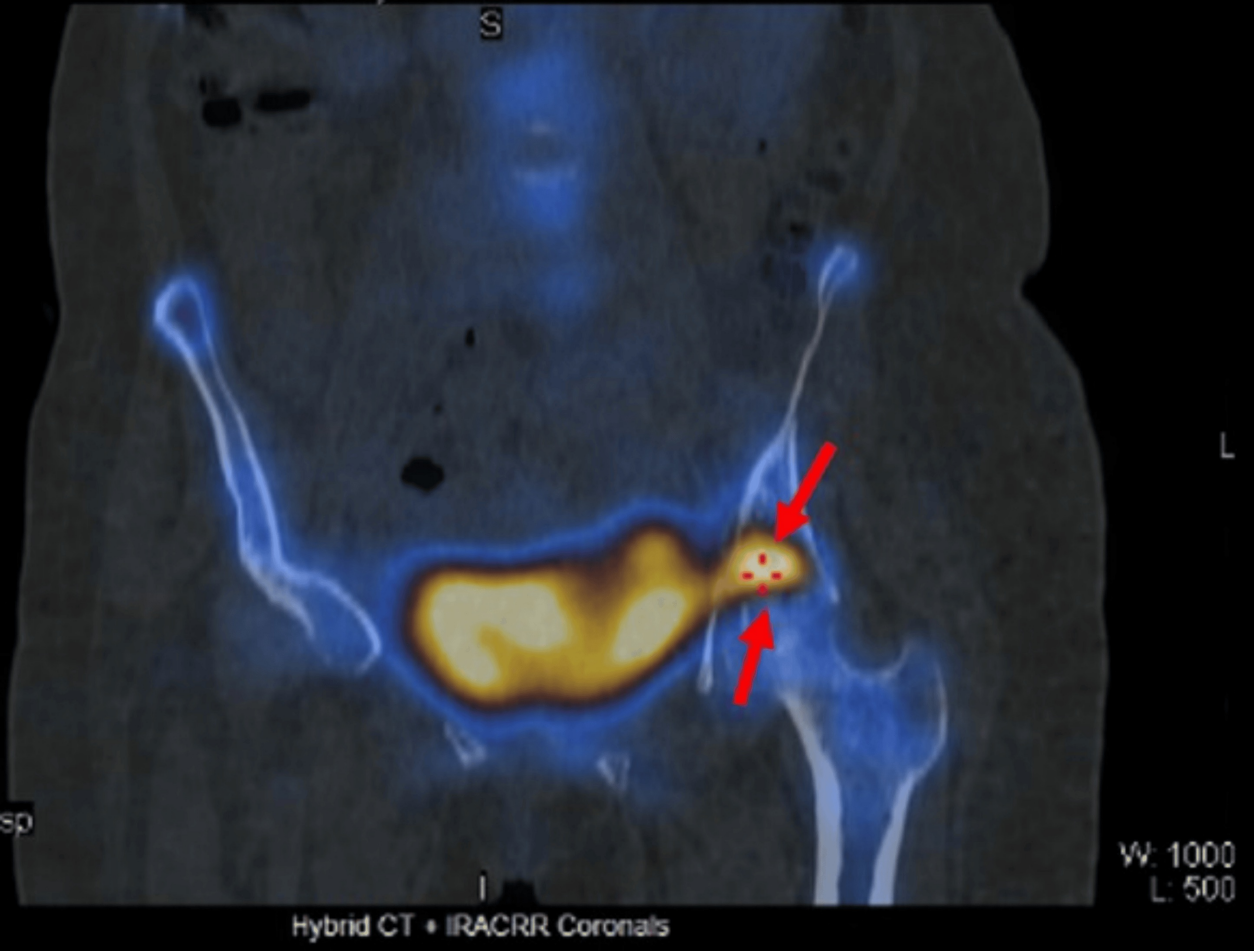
NM bone scan (coronal view) demonstrating a large osteoblastic metastatic lesion in the left acetabulum (arrows). NM bone scan: nuclear medicine bone scan (also known as a bone scintigraphy)
